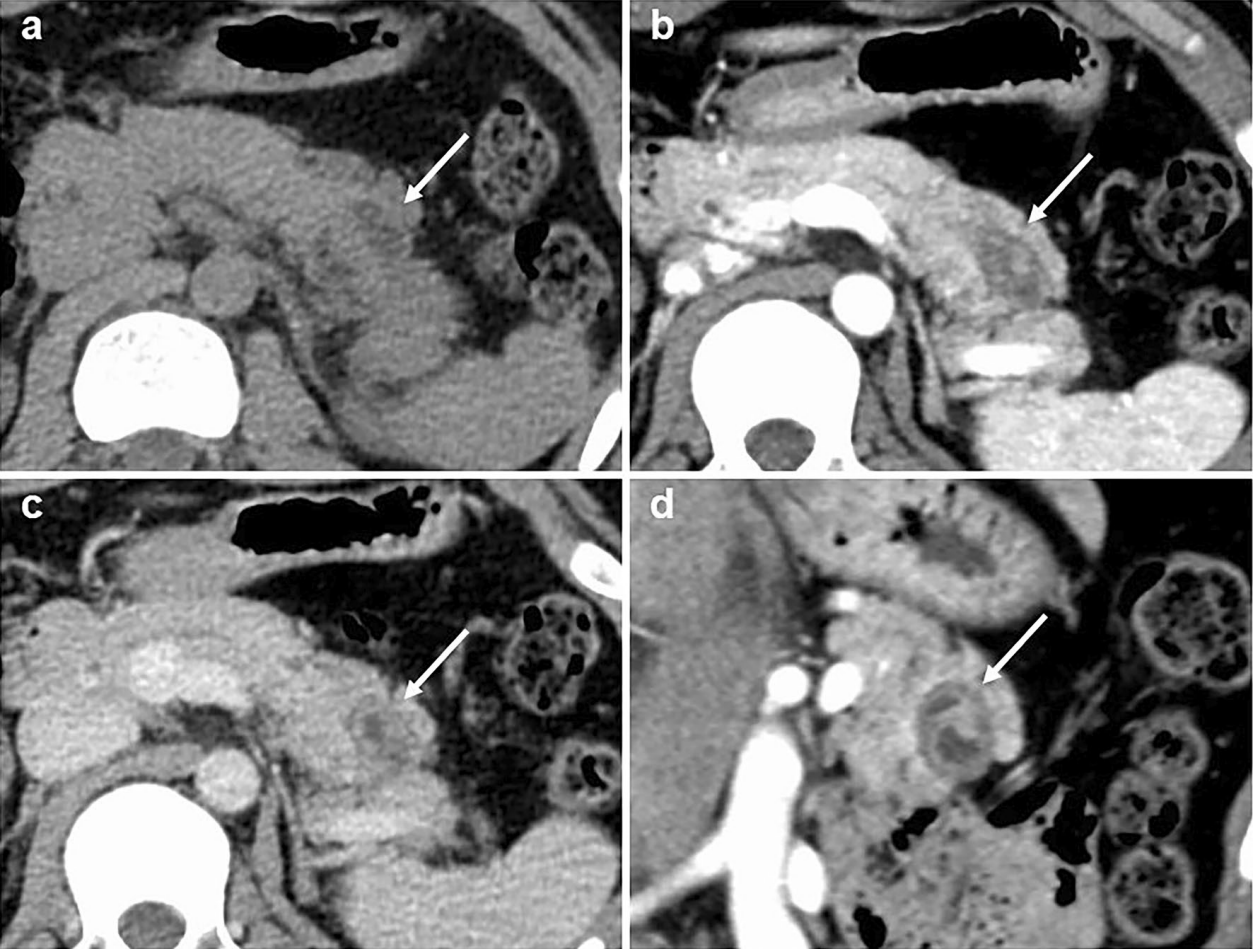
Computed tomography after remission of acute pancreatitis. A 20-mm oval cystic lesion with a thick capsule that enhances the luminal surface was observed in the pancreatic tail (arrow). (a plain phase, b arterial phase, c portal phase, d coronal portal phases)
